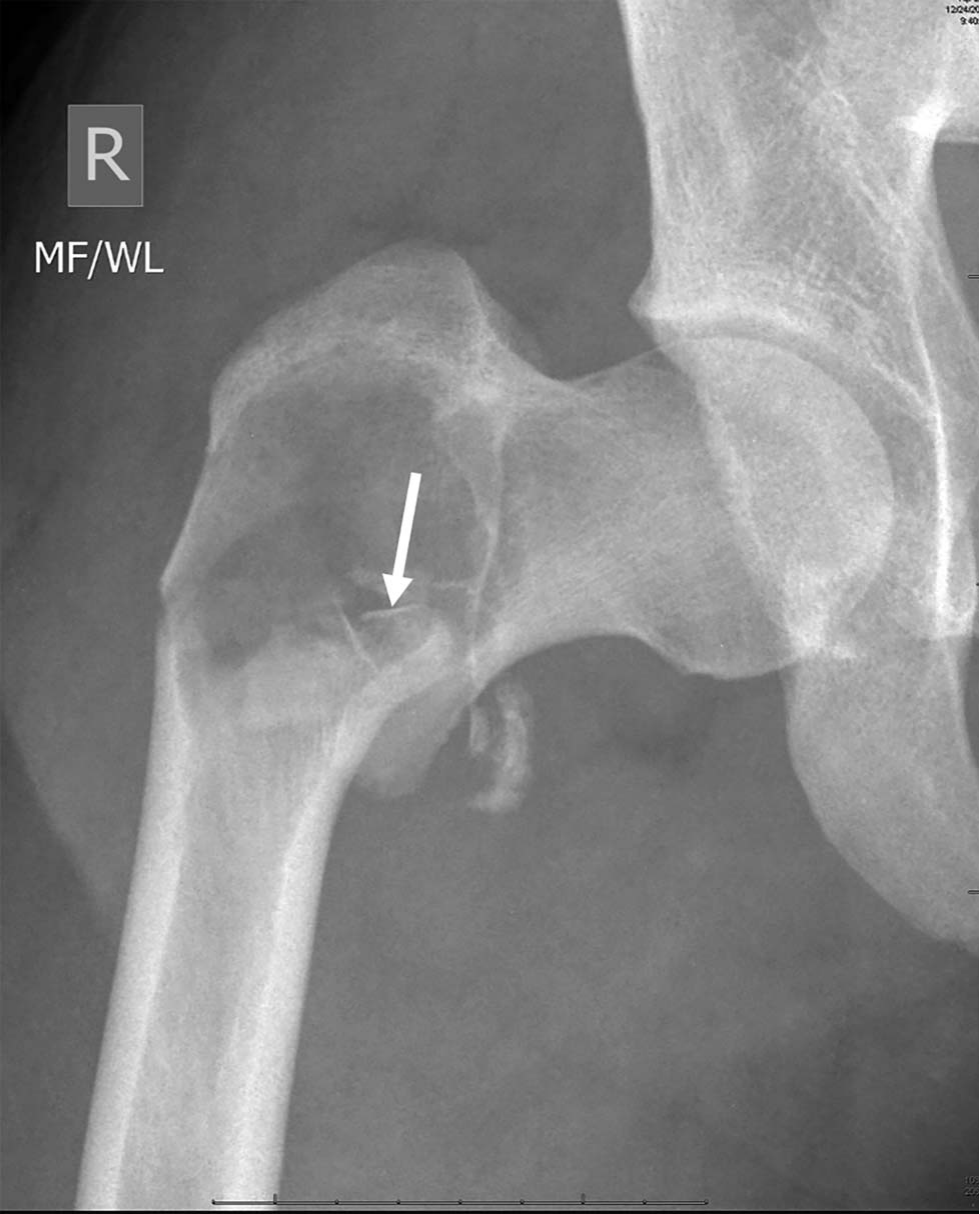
AP radiograph of the right hip at presentation demonstrates a pathological
